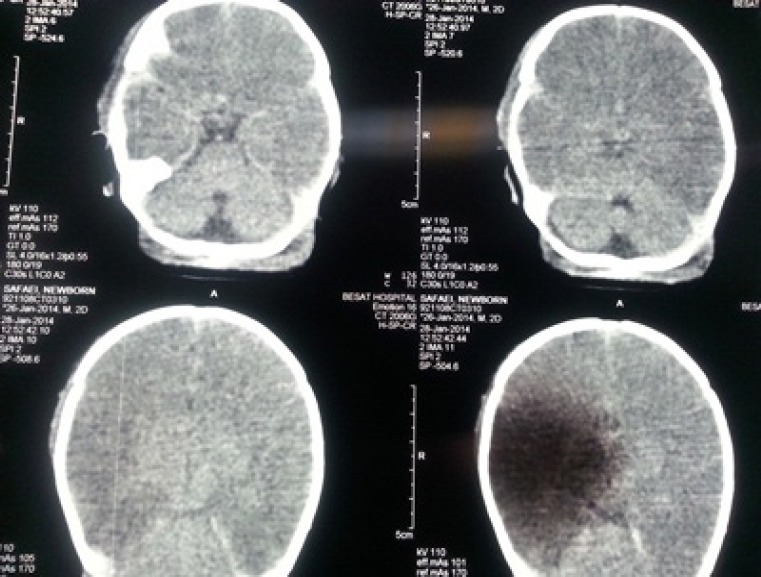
Unenhanced CT, performed on day 2, demonstrates a hypo dense area in the middle part of the right middle cerebral artery territory in the right hemisphere. The right parietal soft tissue swelling was seen. Gray-white matter differentiation was lost diffusely in favor of hypoxemic-ischemic injury.
